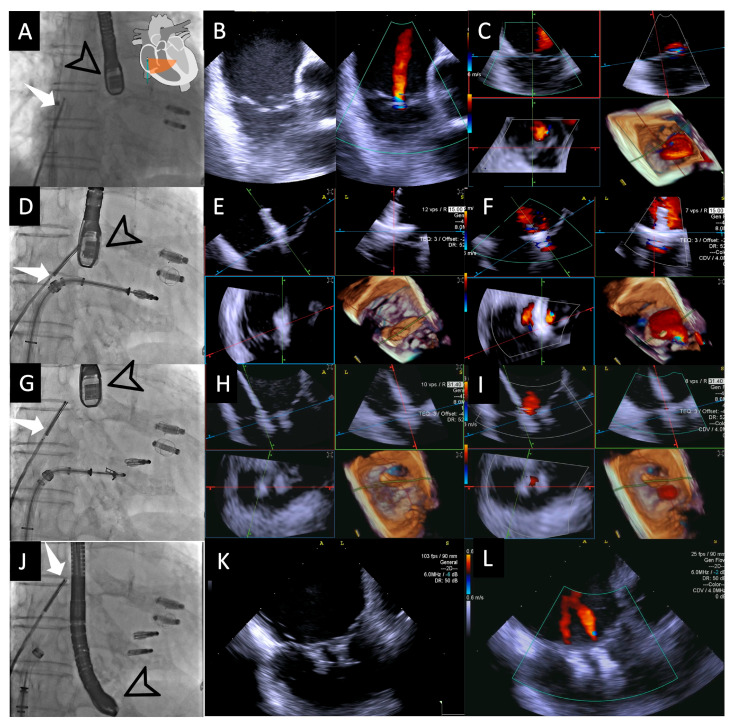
Fluoroscopic and intracardiac views during T-TEER. Fluoroscopic image (A) showing both the 4D-ICE probe (white arrow) and TEE probe (black arrow); the two previously implanted M-TEER devices are visible in this patient. In this case, the ICE probe is positioned directly facing the tricuspid valve, allowing for the first evaluation of the valve through 2D images without and with color Doppler (B), followed by MPR (C), which will serve as our “Home View” throughout the entire procedure. Fluoroscopic image (D) showing the implantation of the first T-TEER device, which is also visualized in MPR as the leaflets are grasped, with clip stability assessed without color (E) and residual jets evaluated with color Doppler (F). Fluoroscopic image (G) showing the implantation of the second edge-to-edge device monitored again through MPR without color (H) and with color Doppler (I). Fluoroscopic image (J) during the final evaluation of results, with a 2D image without color confirming good device stability (K) and a 2D image with color Doppler demonstrating mild residual regurgitant jets (L). Abbreviations: 4D-ICE: 4D intracardiac echocardiography. MPR: multiplanar reconstruction. M-TEER: mitral transcatheter edge-to-edge repair; T-TEER: tricuspid transcatheter edge-to-edge repair.
